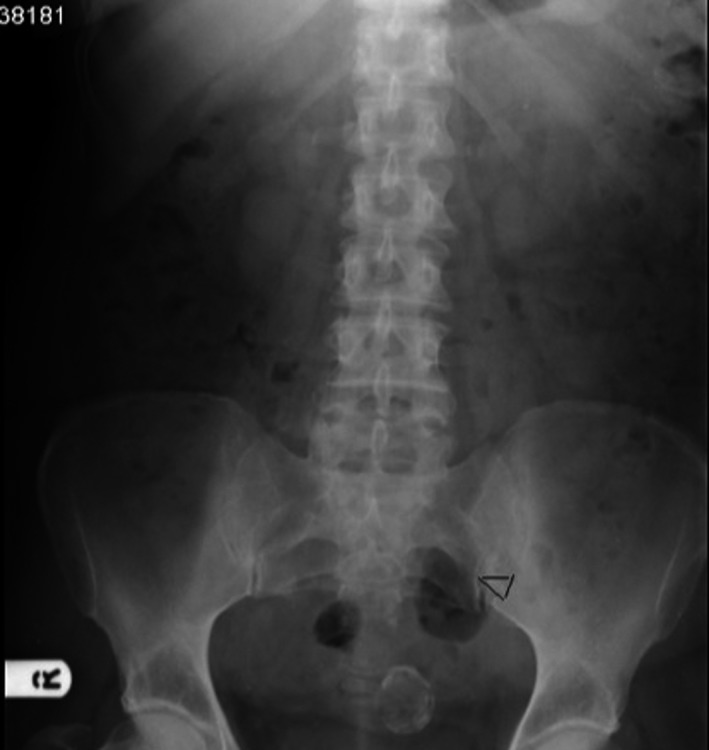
Follow‐up abdominal radiograph.
